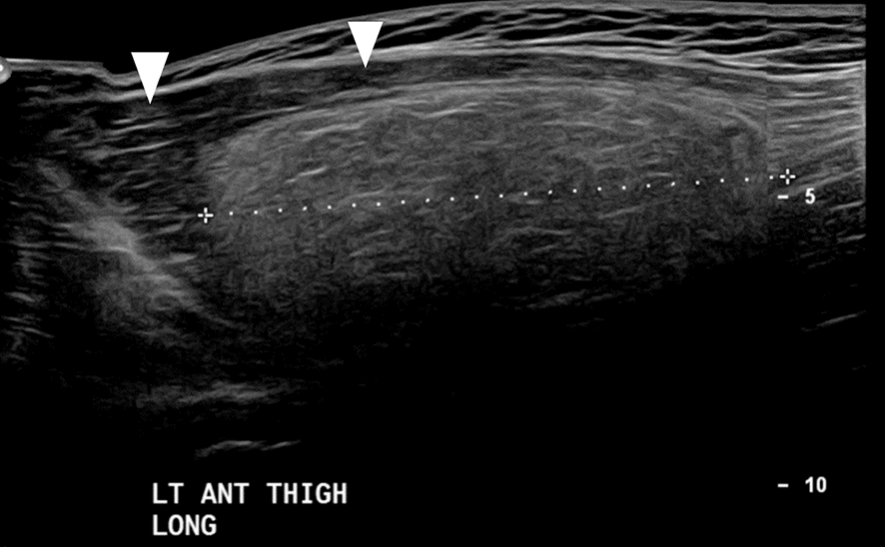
Long axis ultrasound scan of the left thigh demonstrating a large
